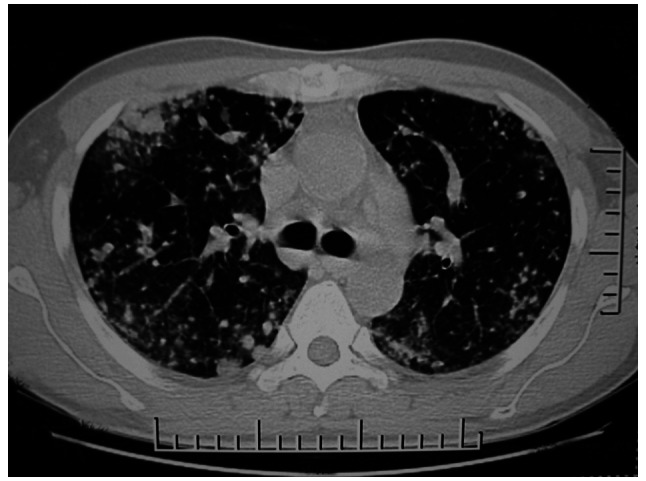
The computed tomography (CT) of the chest shows that the multiple bilateral nodules progressed rapidly following surgery.
